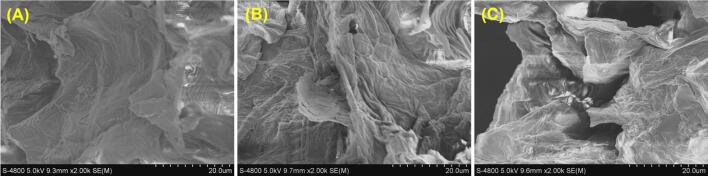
SEM images of sour jujube leaves before treatment (A), after Soxhlet extraction (B), and after ultrasound-assisted extraction (C).
